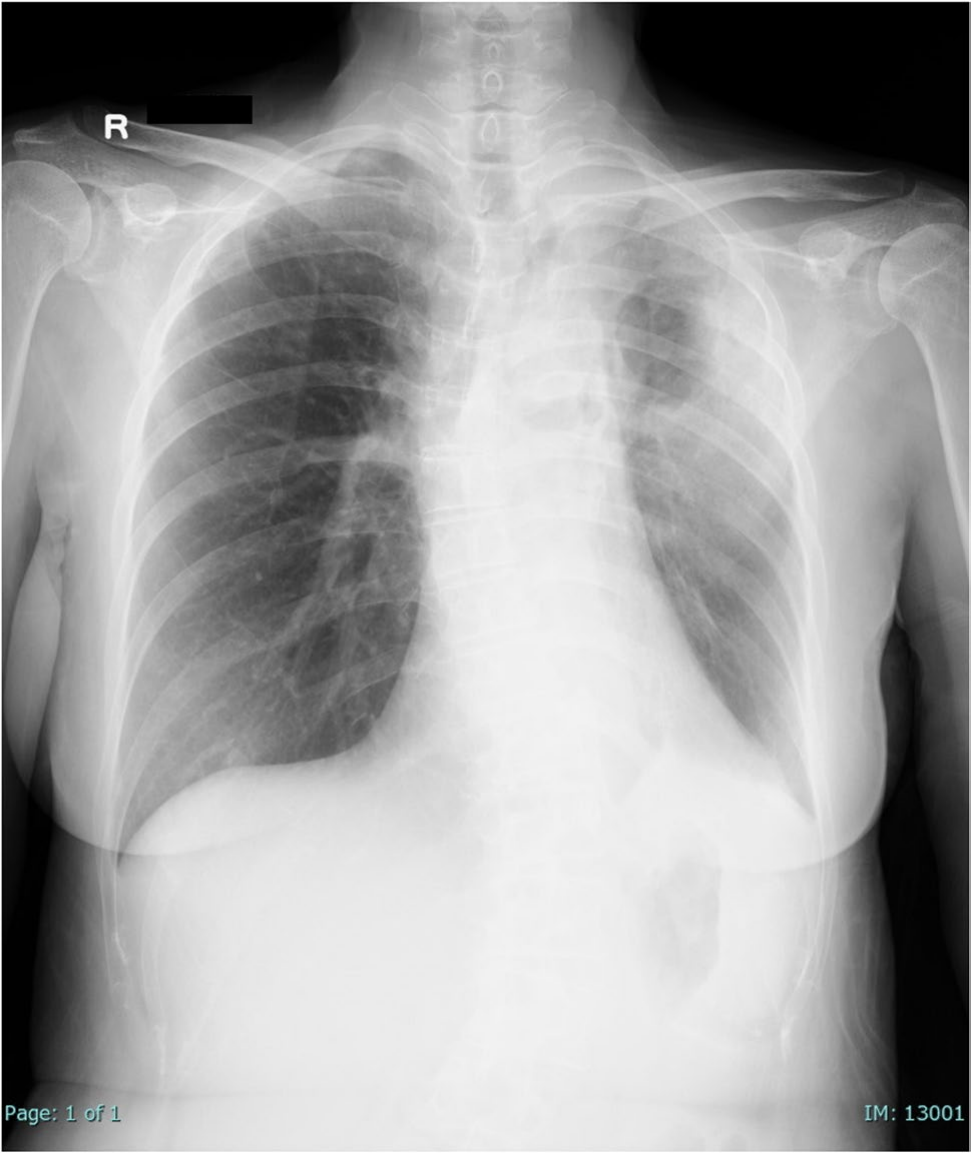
Chest X-ray image at admission, clearly showing infiltrative shadowing in the left lung
